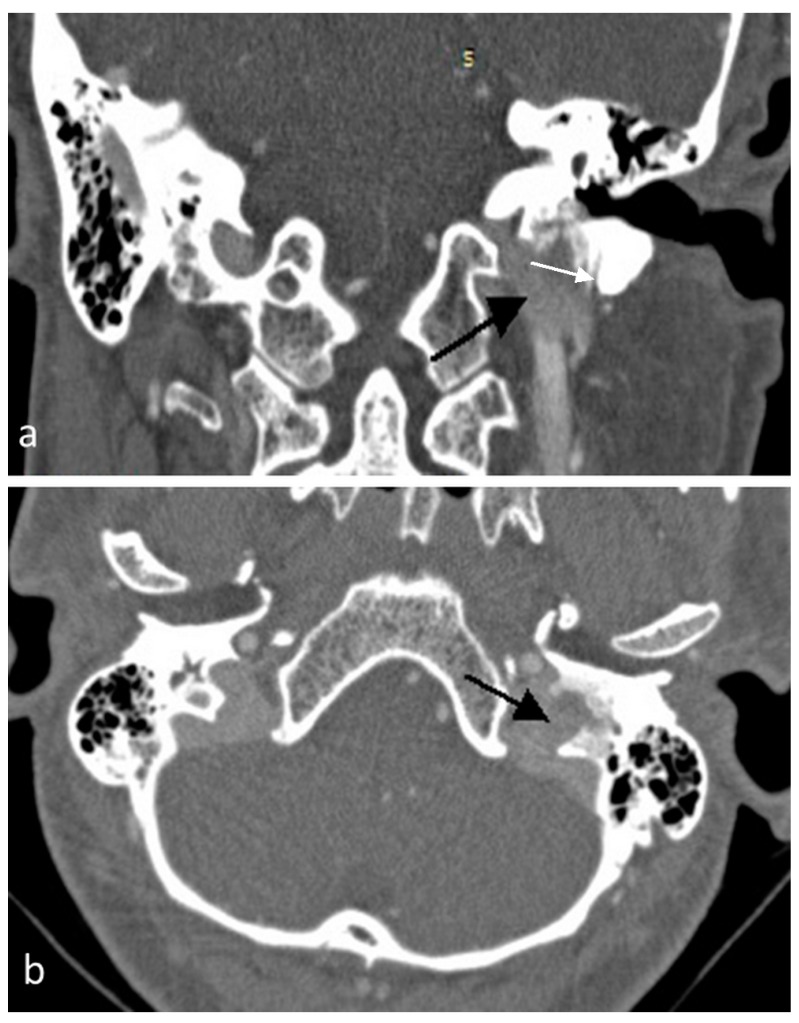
Computed tomography scan of temporal bone, (a) coronal and (b) axial views show soft tissue mass destructing the jugular foramen (black arrow). White arrow indicates stylomastoid foramen.
